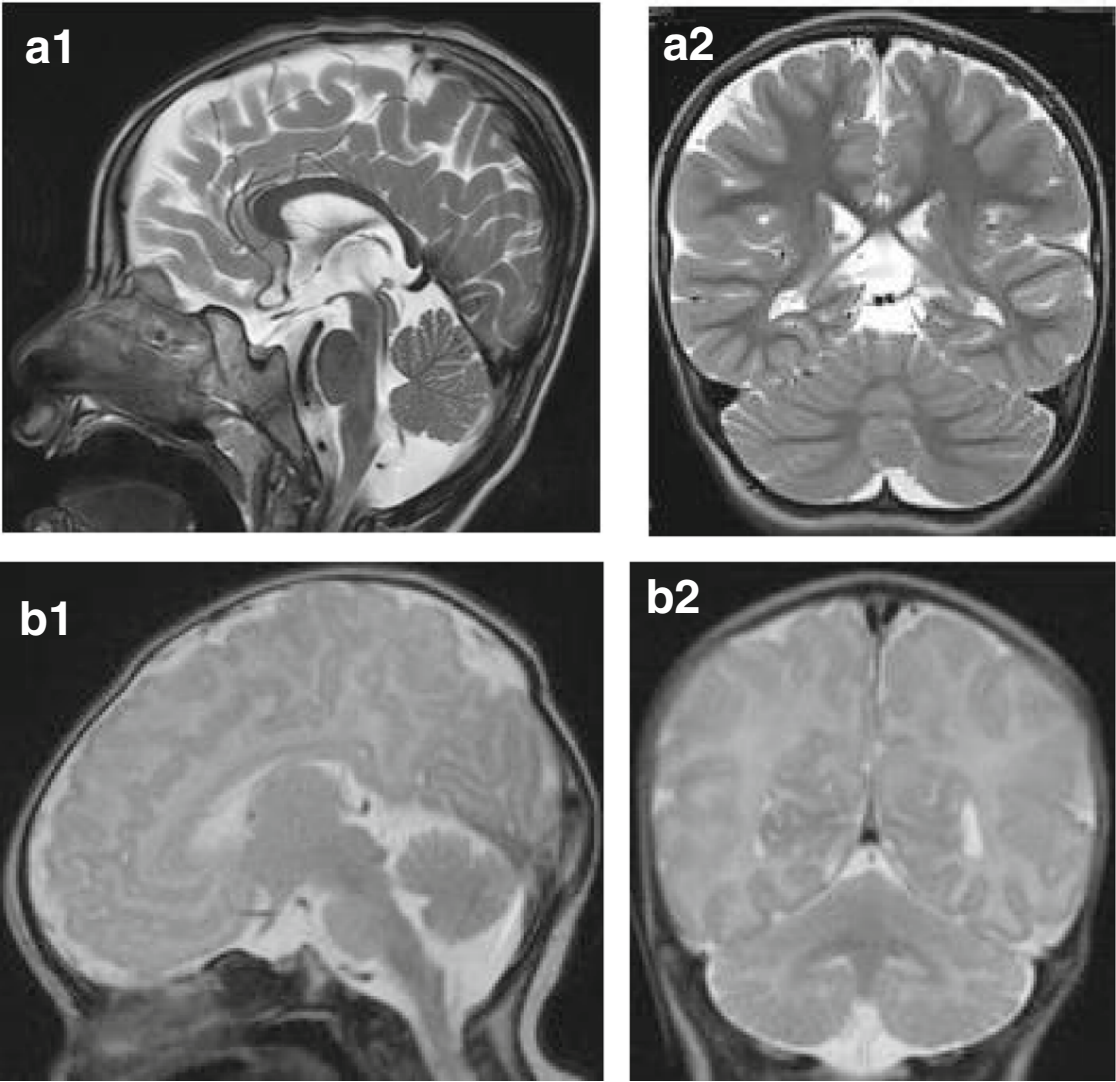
Brain MRI T2-weighted, sagittal (1) and coronal (2) sections. a
